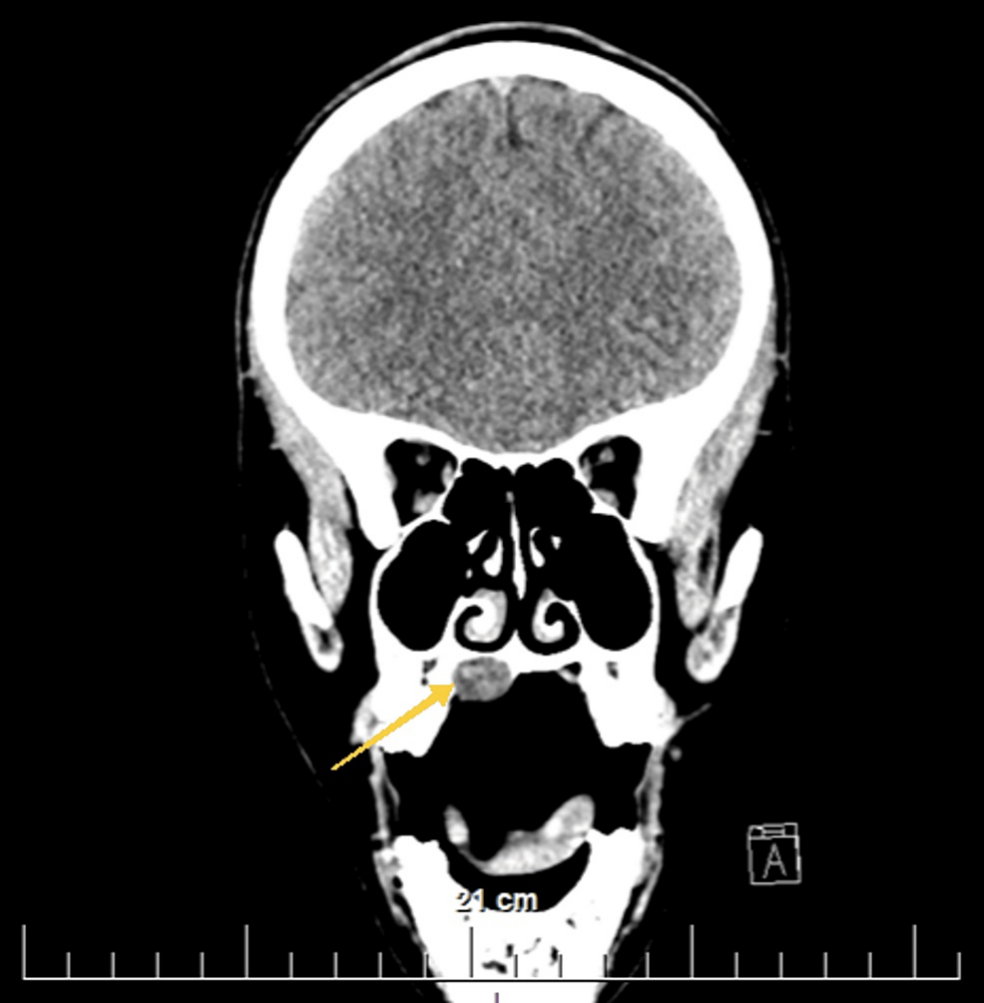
Transverse section of a native head CT. A yellow arrow indicates the location of the hard palate lesion on the right
